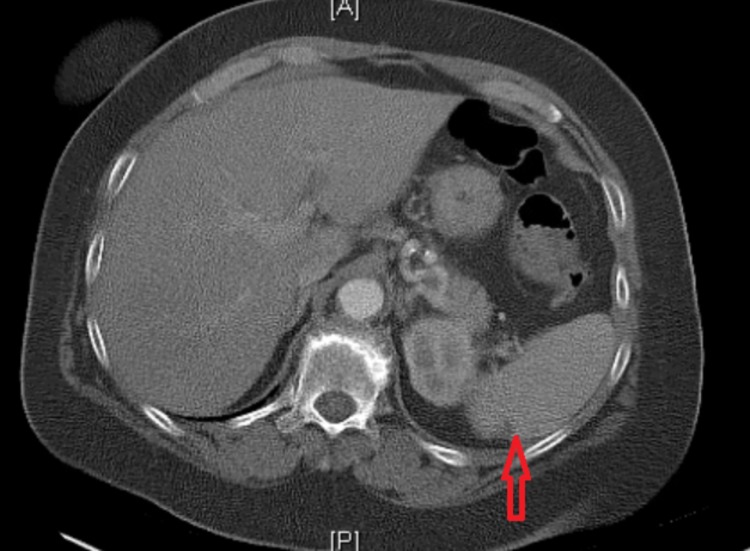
Computed tomography (CT) scan of the abdomen showing a splenic infarct
